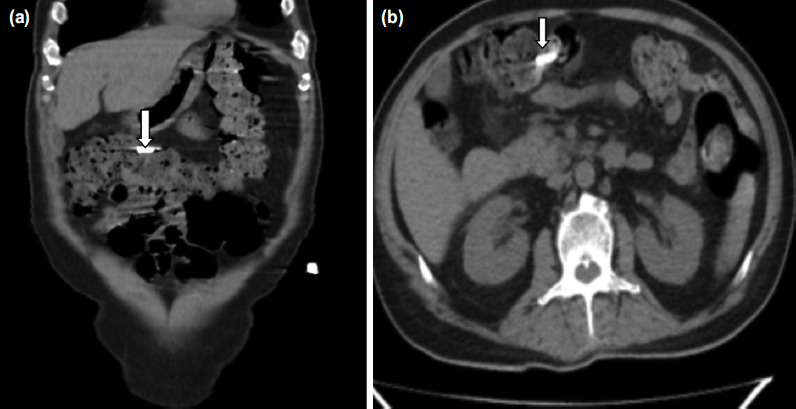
(a) Showing coronal cut of abdominal CT scan which shows the migrated screw from cervical plate as pointed with white arrow, (b) showing cross-sectional cut of CT scan with migrated screw marked with a white arrow.
